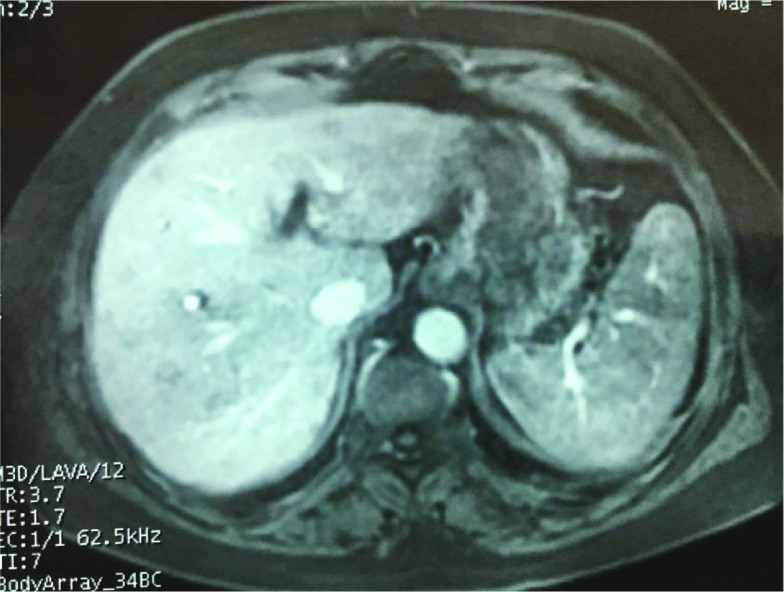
Contrast enhanced T1-weighted sequence on MRI showing very week enhancement.
